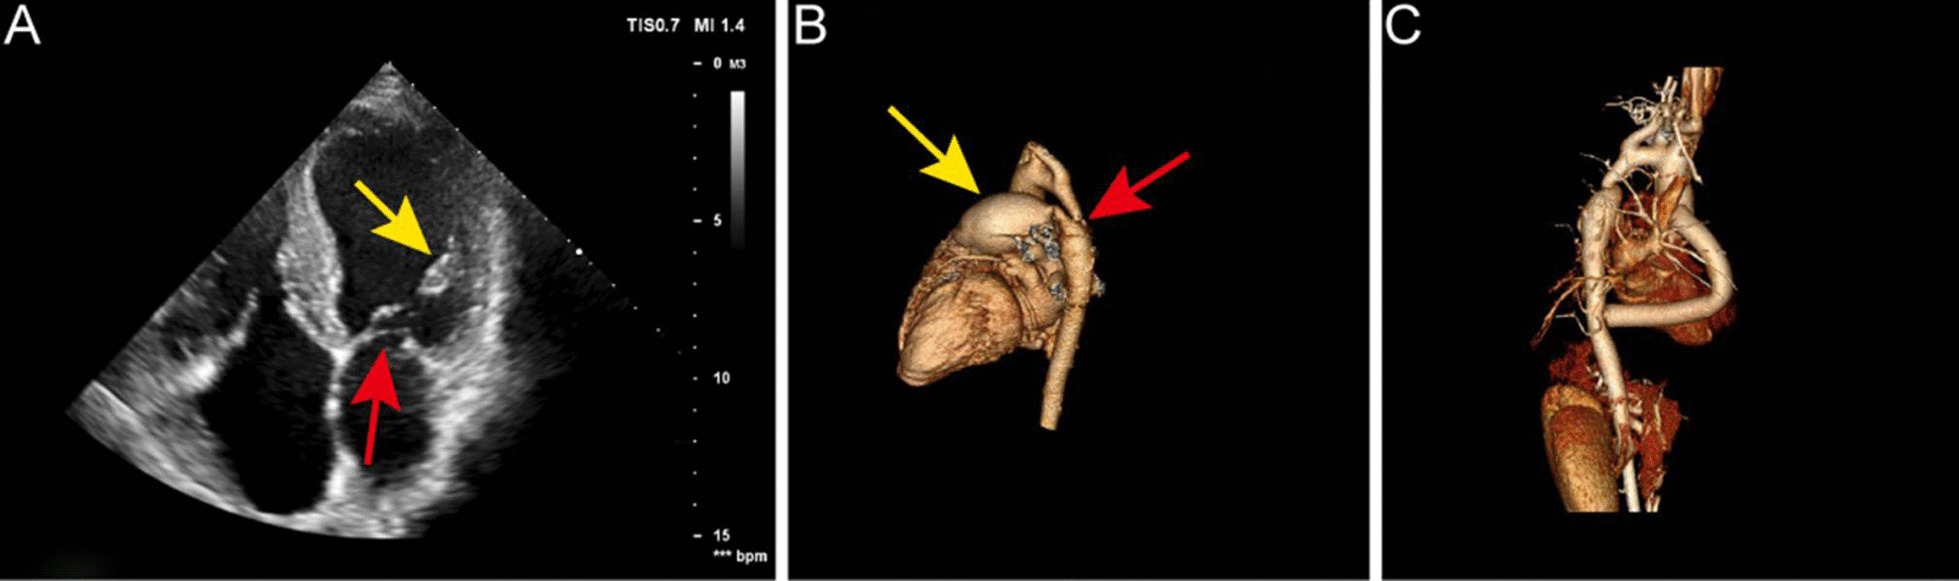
Echocardiography and CT examination reports. A Supravalvular mitral membrane attached to the wall of the left atrium (red arrow) and solitary papillary muscle (yellow arrow). B CT of patent ductus arteriosus. A localized narrowing was observed at the aortic arch near the descending aorta (red arrow) and pulmonary artery dilatation (yellow arrow). C Prosthetic bypass between the ascending and the descending aorta
